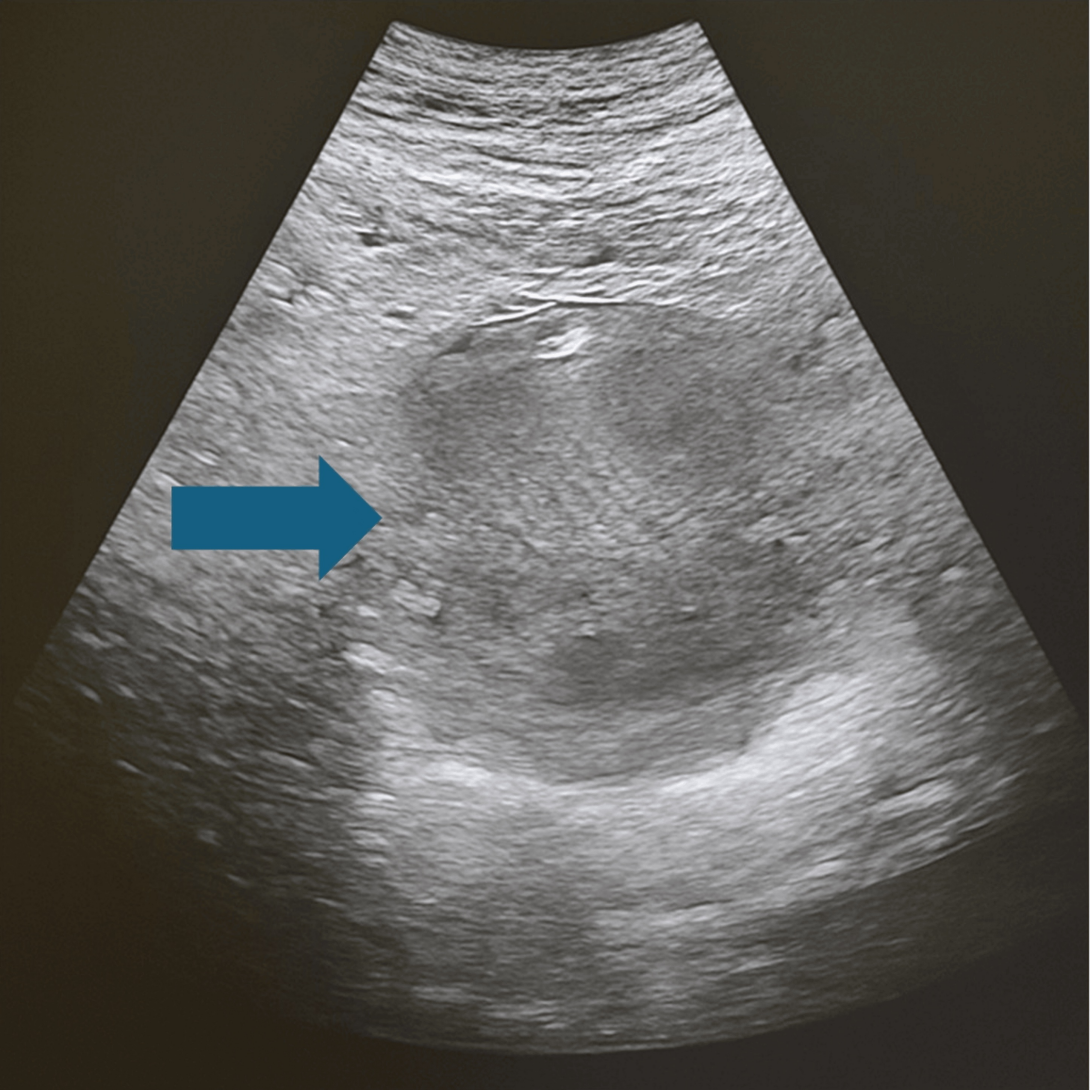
Ultrasound image demonstrating a markedly enlarged uterus with a hypoechogenic intrauterine fluid collection consistent with pyometra (arrow) No evidence of free peritoneal fluid was observed, arguing against uterine perforation or associated peritonitis.
